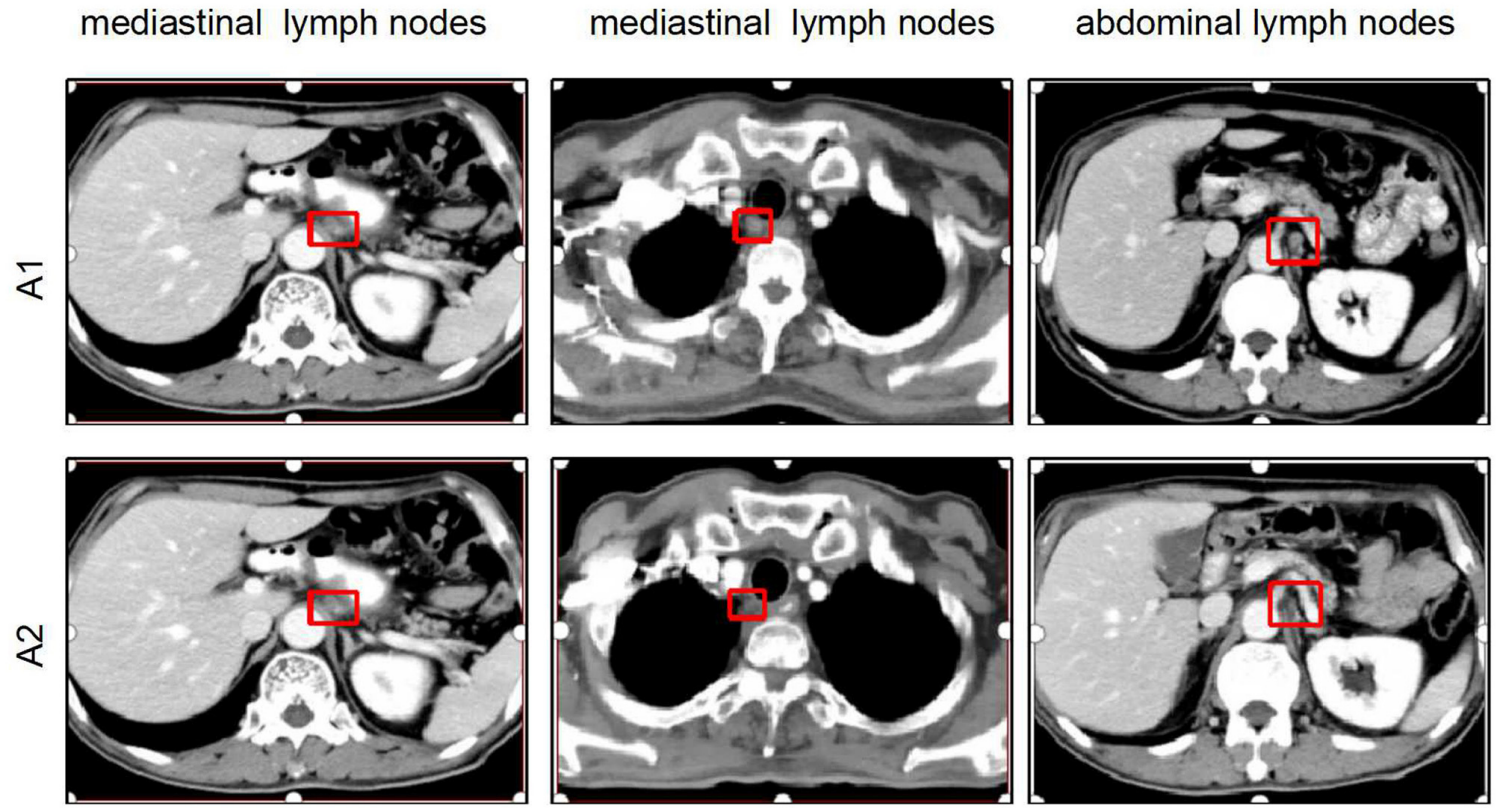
Comparison of chest CT scan between before and after treatment for the first patient. (A1) Chest CT scan showing enlarged lymph nodes in the retroperitoneum and mediastinum in postoperative re-examination. (A2) Chest CT showing that the enlarged lymph nodes in the retroperitoneum and mediastinum shrank or became loosened after 6 weeks of anlotinib combined chemoradiotherapy. CT, computed tomography; PD, progressive disease; PR, partial progressive disease.
